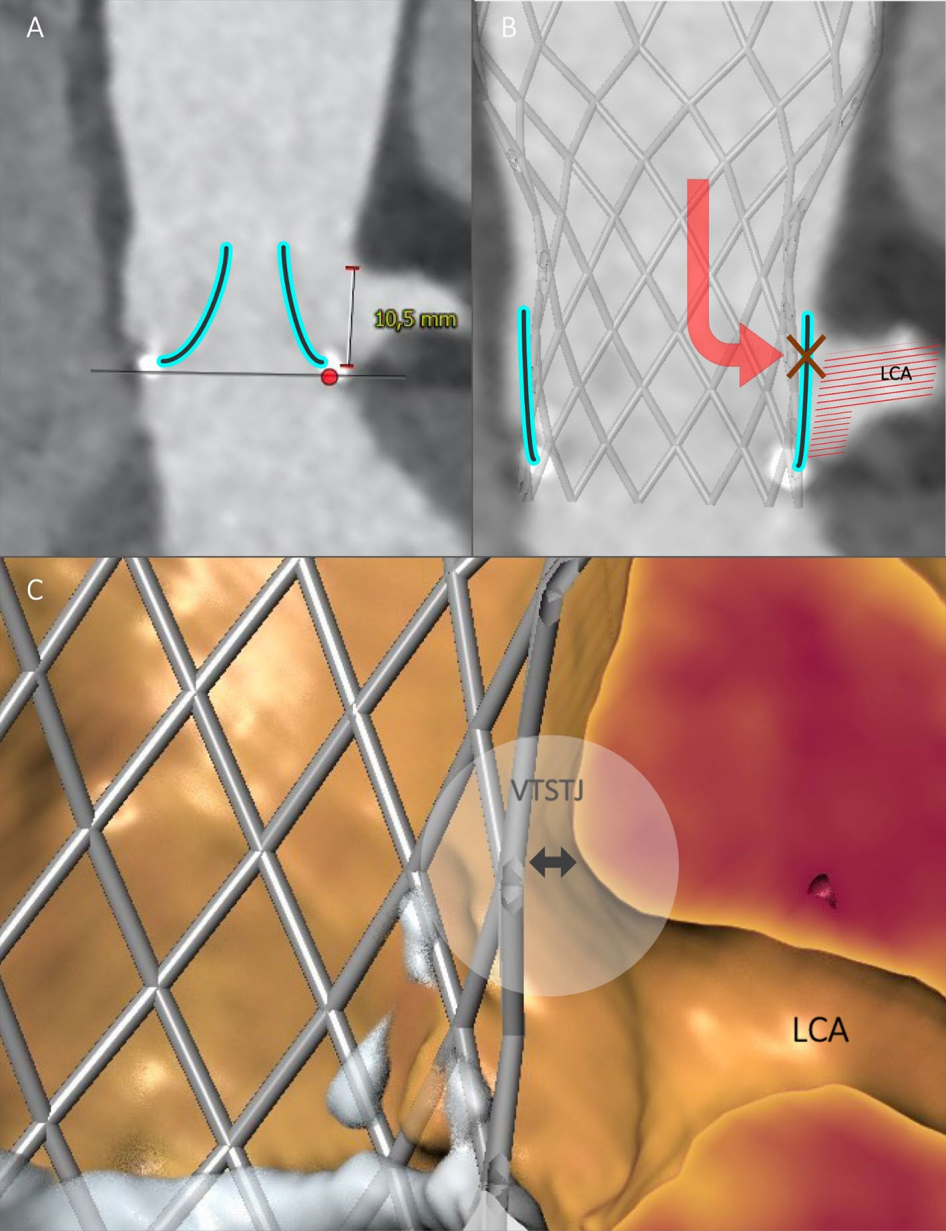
Sequestered Sinus. A Sequestered Sinus (pre-procedural CT). Sequestered Sinus is defined by low STJ height (indicated measurement, 10.5 mm) and narrow STJ diameter. Surgical valve leaflets have been drawn in retrospectively. B Coronary obstruction in a patient with Sequestered Sinus [pre-procedural CT with a virtually implanted THV (Evolut R, *Medtronic*)]. Following THV implantation the surgical valve leaflet indirectly inhibits blood flow to SOV and LCA by obstruction at the STJ. C Valve-to-sinotubular junction (VTSTJ) distance (3D CT reconstruction). *CT* computed tomography, *LCA* left coronary artery, *SOV* sinus of Valsalva, *STJ* sinotubular junction, *THV* transcatheter heart valve, *VTSTJ* valve-to-sinotubular junction
